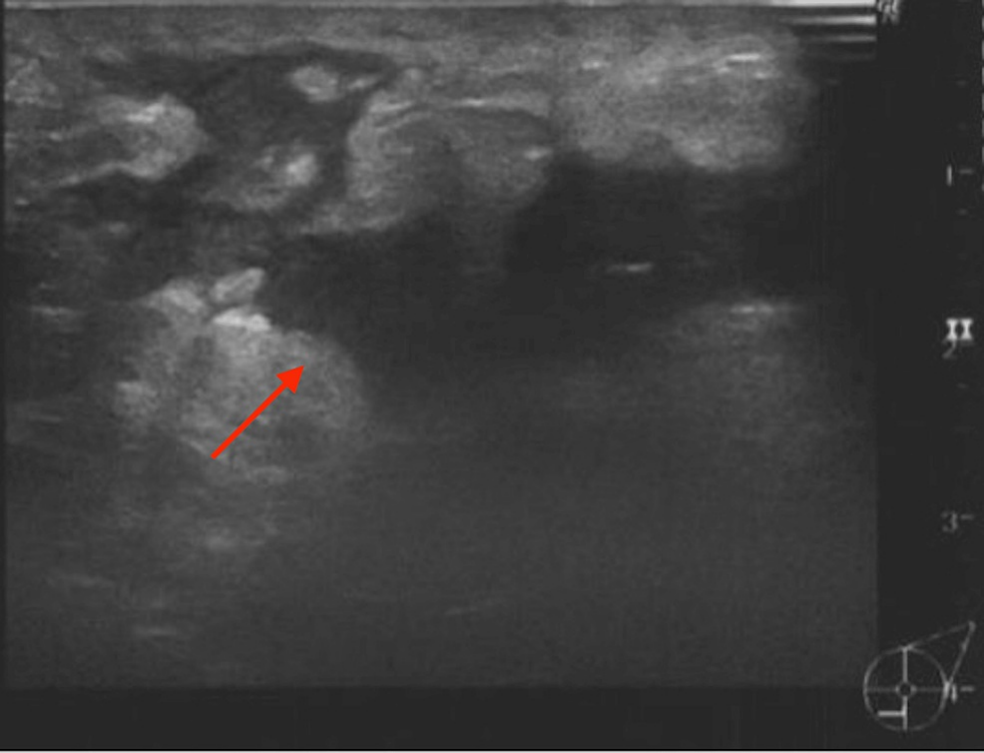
Ultrasonography of the left breast mass. More than 4 cm of the irregular hypoechoic area is observed in the left mammary gland.
